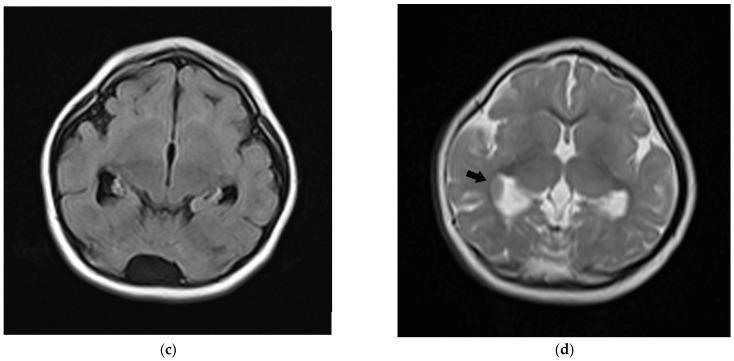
Brain MRI follow-up at 2 years 1 month of age. (a) Spin echo T1, sagittal view: enlarged posterior fossa with large cisterna magna. (b) Inversion recovery, coronal view: posteriorly enlarged lateral ventricles. (c) Fluid-attenuated inversion recovery, axial view: enlarged ventricles and fronto-polar simplified gyral pattern. (d) Turbo spin echo T2, axial view: right periventricular nodular heterotopia (arrow).
